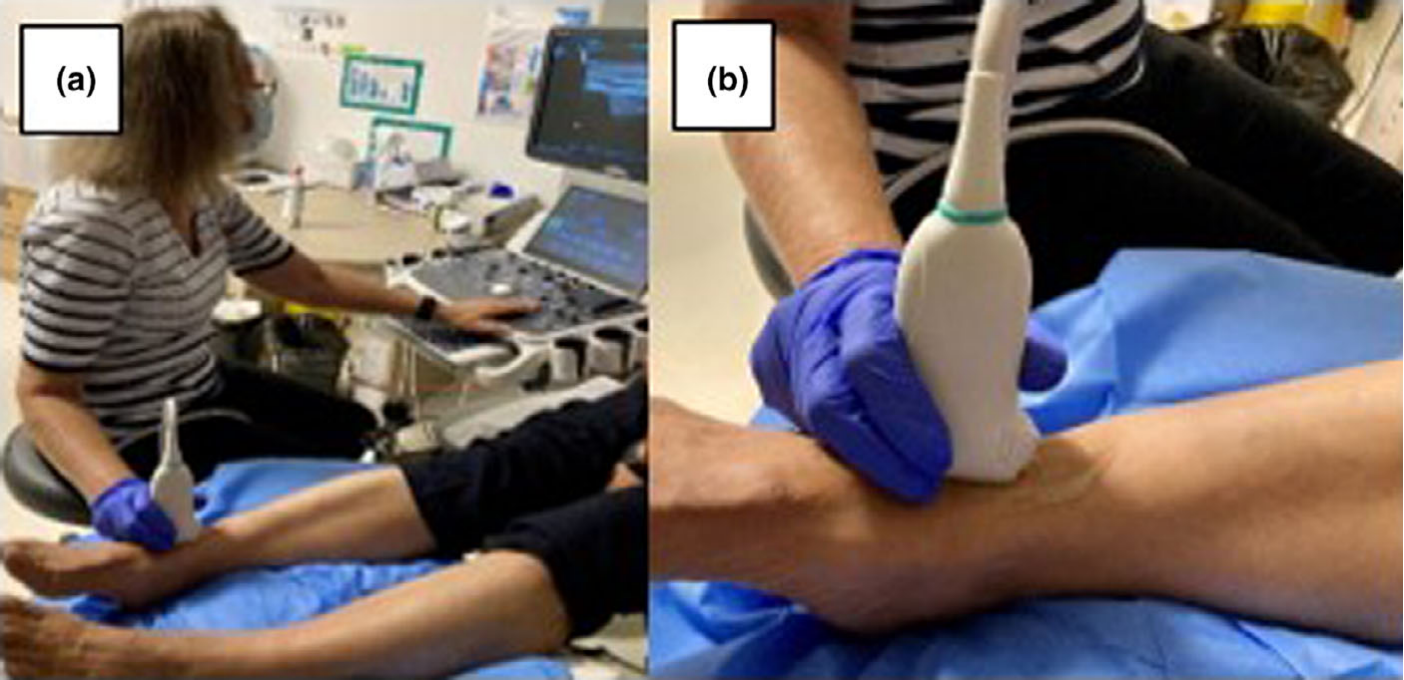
(a) Ultrasound scanning position of the proximal dorsalis pedis artery in longitudinal axis with a 13–3‐MHz linear transducer; (b) transducer positioned at proximal dorsalis pedis artery longitudinally.
